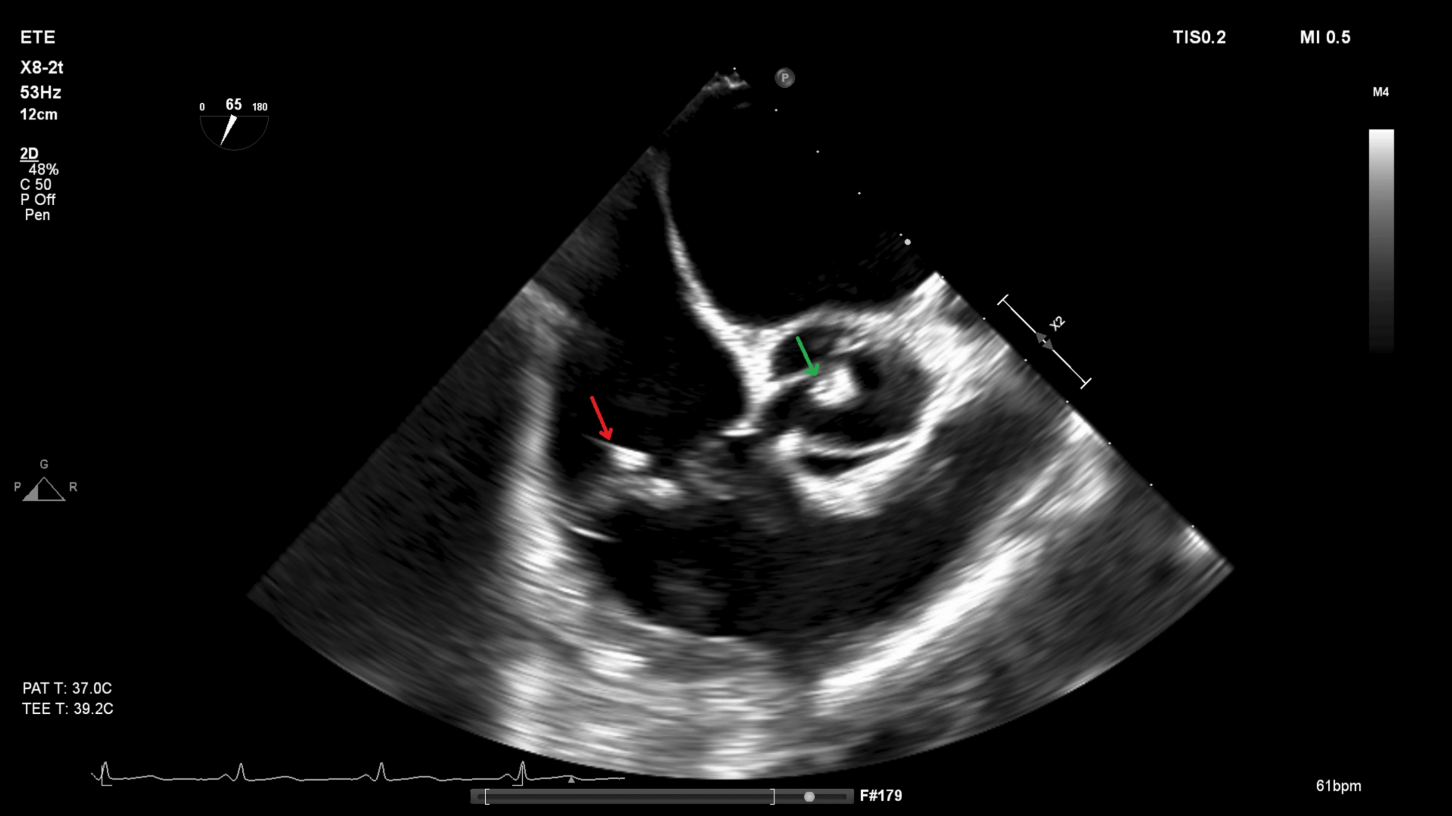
Transesophageal echocardiogram (short-axis view) showing vegetations on the tricuspid valve (red arrow) and the aortic valve (green arrow), which are the cause of the ischemic stroke.
